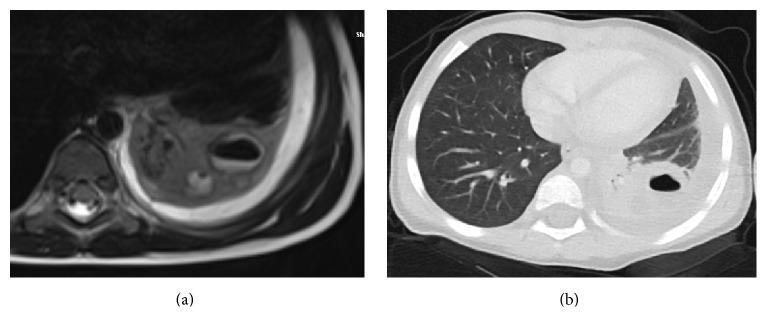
Thoracic vertebrae MR (a) and thorax CT (b) images: necrotizing pneumonia in the left lower lobe of lung.
